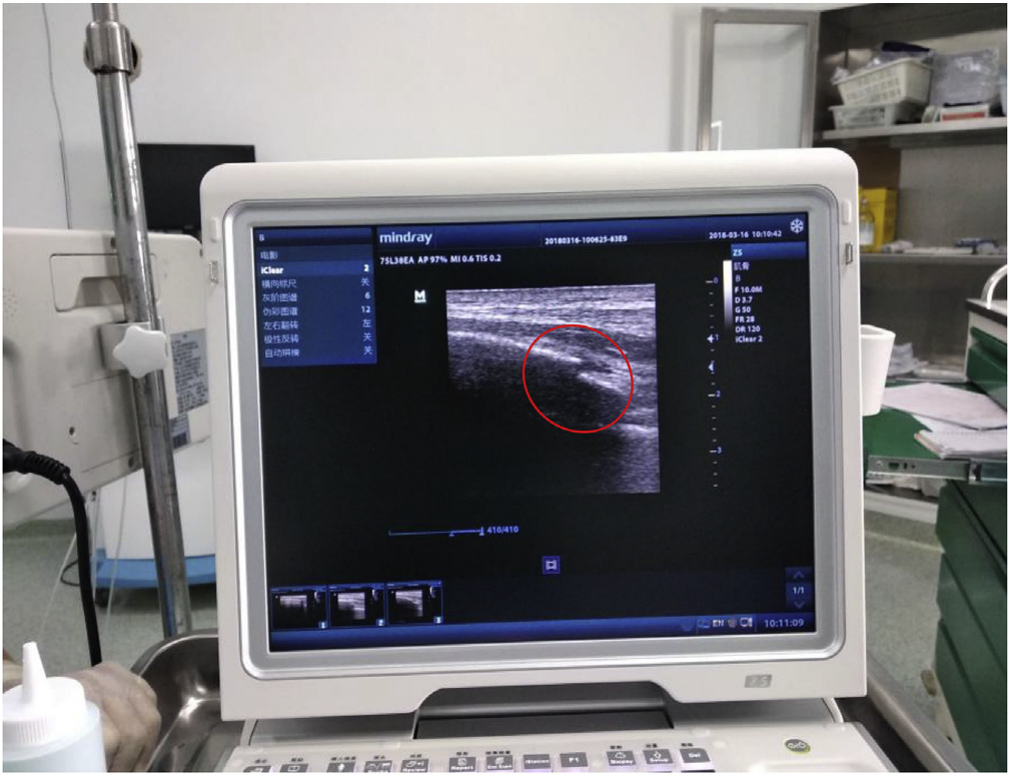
Ultrasound Image localizing rib fracture.
